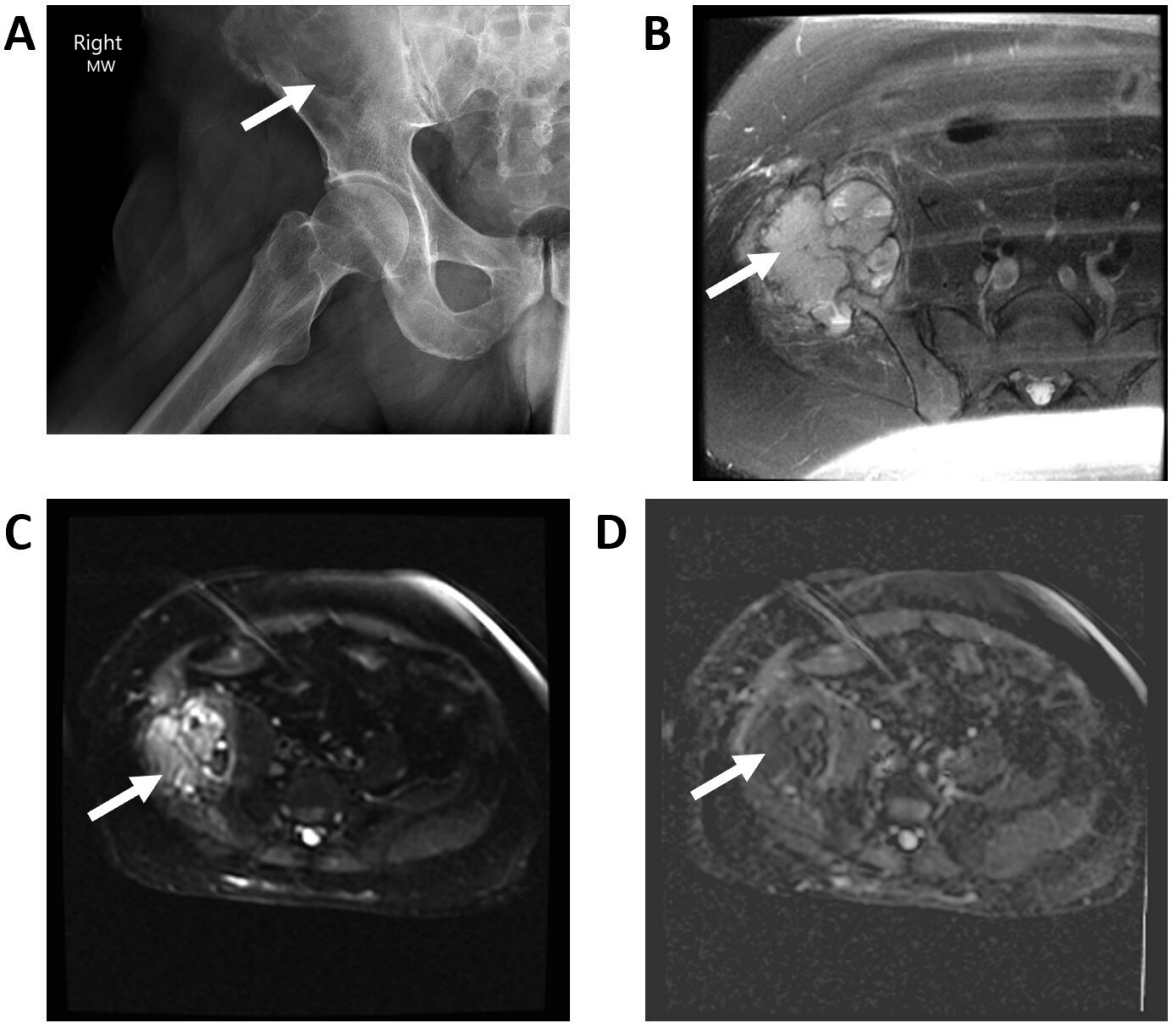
Multiple myeloma of ilium (arrows): (A) pelvis X-ray, (B) fs*T*
